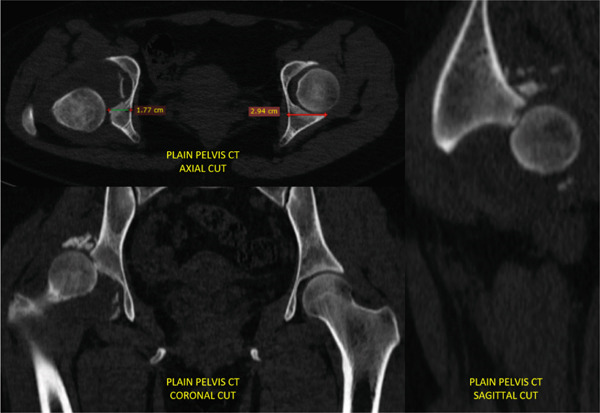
Case 4 preoperative computed tomography.
